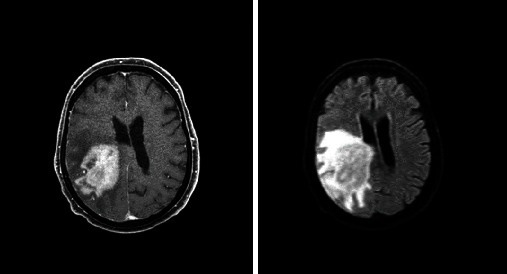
Enhancing mass in the right parietal lobe with vasogenic edema extending into the right posterior frontal lobe and right temporal lobe. Radiographic differential diagnosis: glioblastoma.
